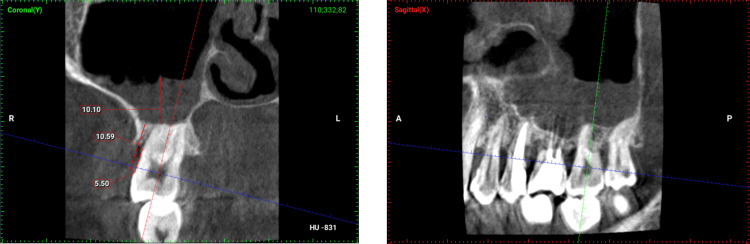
Coronal and sagittal sections of CBCT radiograph with measurements (R) right side, (L) left side, (A) anterior, (P) posterior CBCT – cone-beam computerized tomography
